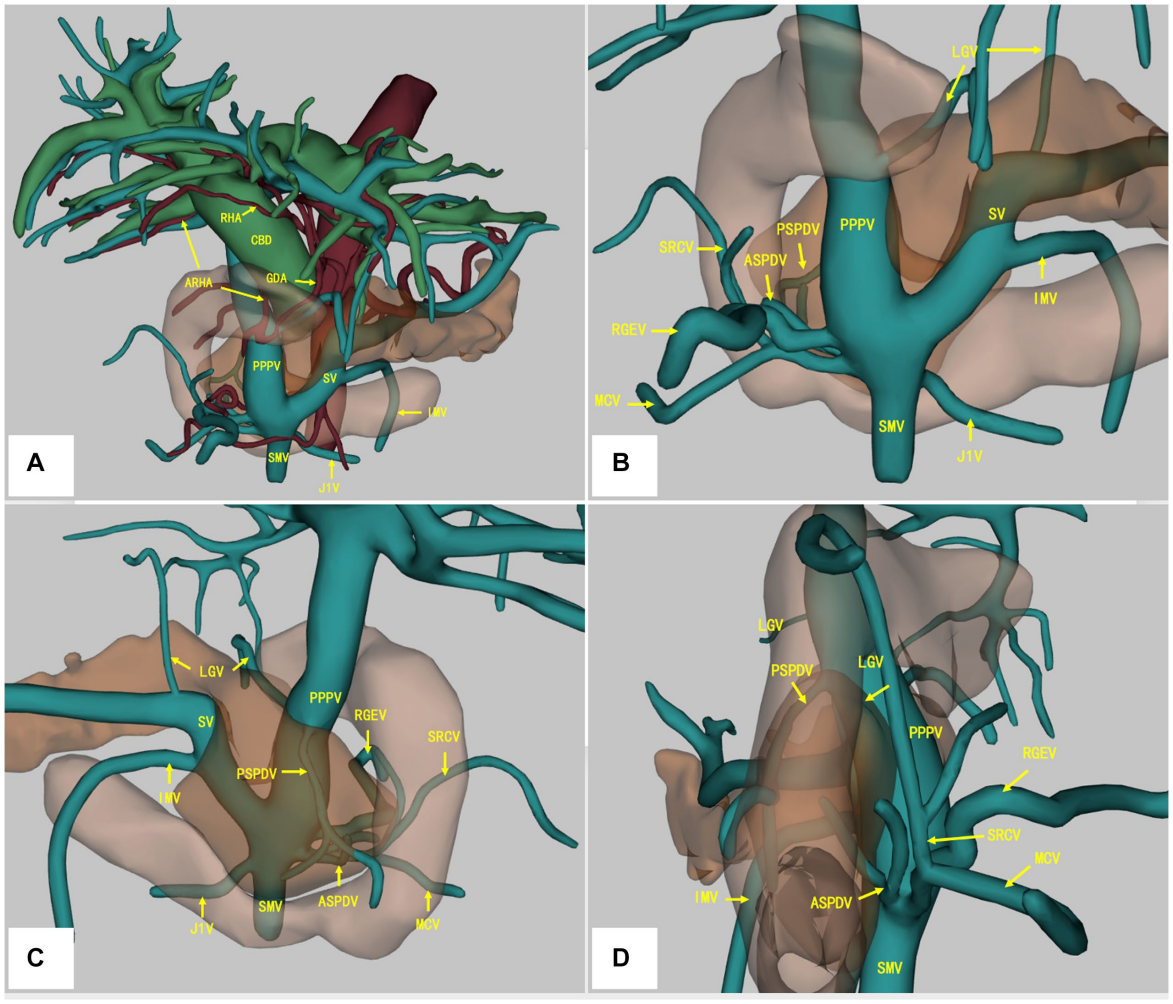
Three-dimensional vascular reconstruction of contrast-enhanced CT scan. The PPPV was located anterior to the pancreas and posterior to the duodenum, and the L-shaped SV was longitudinally located posterior to the pancreatic neck (A). The RHA was located anterior to the CBD, and the ARHA originated from GDA was located posterior to the PV (A). The branches of PV/SMV system were depicted from the anterior (B), posterior (C), and right side (D). PPPV, prepancreatic postduodenal portal vein; SV, spleen vein; SMV, superior mesenteric vein; LGV, left gastric artery; PSPDV, posterior superior pancreaticoduodenal vein; ASPDV, anterior pancreaticoduodenal superior pancreaticoduodenal vein; SRCV, superior right colonic vein; RGEV, right gastroepiploic vein; MCV, middle colon vein; J1V, the first jejunal vein; RHA, right hepatic artery; ARHA, accessory right hepatic artery; GDA, gastroduodenal artery; CBD, common bile duct.
